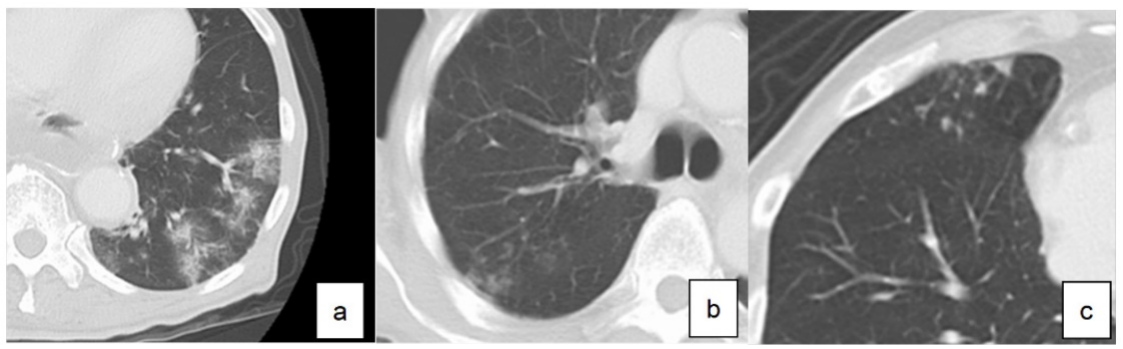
Definition of diagnosis of pneumonia based on computed tomography. a. consolidation type, b. reticular type, c. nodular type
